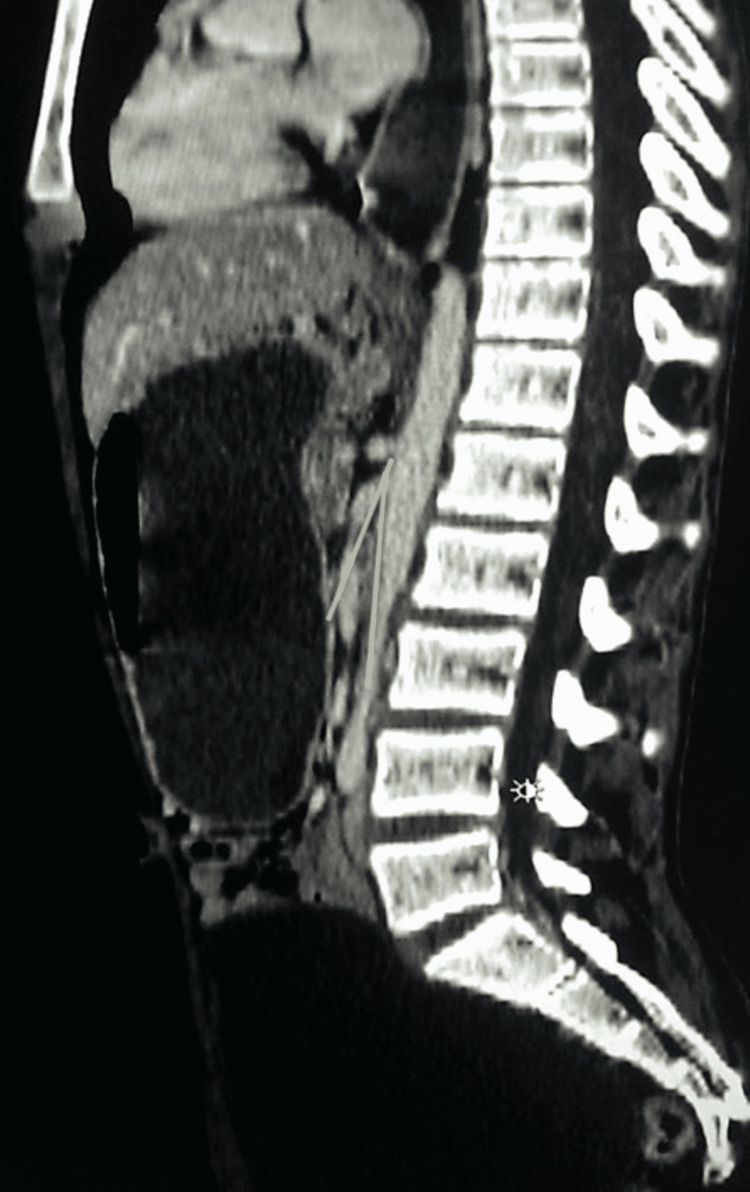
Computed tomography scan of the abdomen in sagittal view with two yellow angled lines showing the reduced aorto-mesenteric angle.
